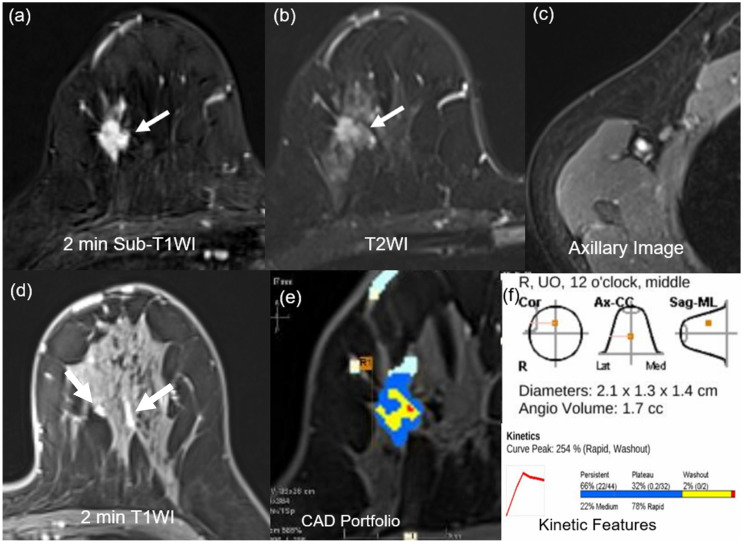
MR images of a 40-year-old woman with a 2.1-cm surgically verified invasive ductal breast cancer and germline BRCA-negativity (a) Axial contrast-enhanced T1-weighted 2 min subtraction image shows an irregular, spiculated, heterogeneously enhancing mass in the right breast (arrow). (b) The T2-weighted image shows no peritumoral edema near the mass (arrow). (c) Axillary image shows no axillary adenopathy. (d) Axial contrast-enhanced T1-weighted 2 min after contrast enhancement image shows moderate background parenchymal enhancement (arrows). (e,f) An auto-portfolio of the CAD system demonstrated 2.1 cm in the largest tumor size and 1.7 cc in angio-volume above the enhancement threshold. Delayed-washout component was 2%.
